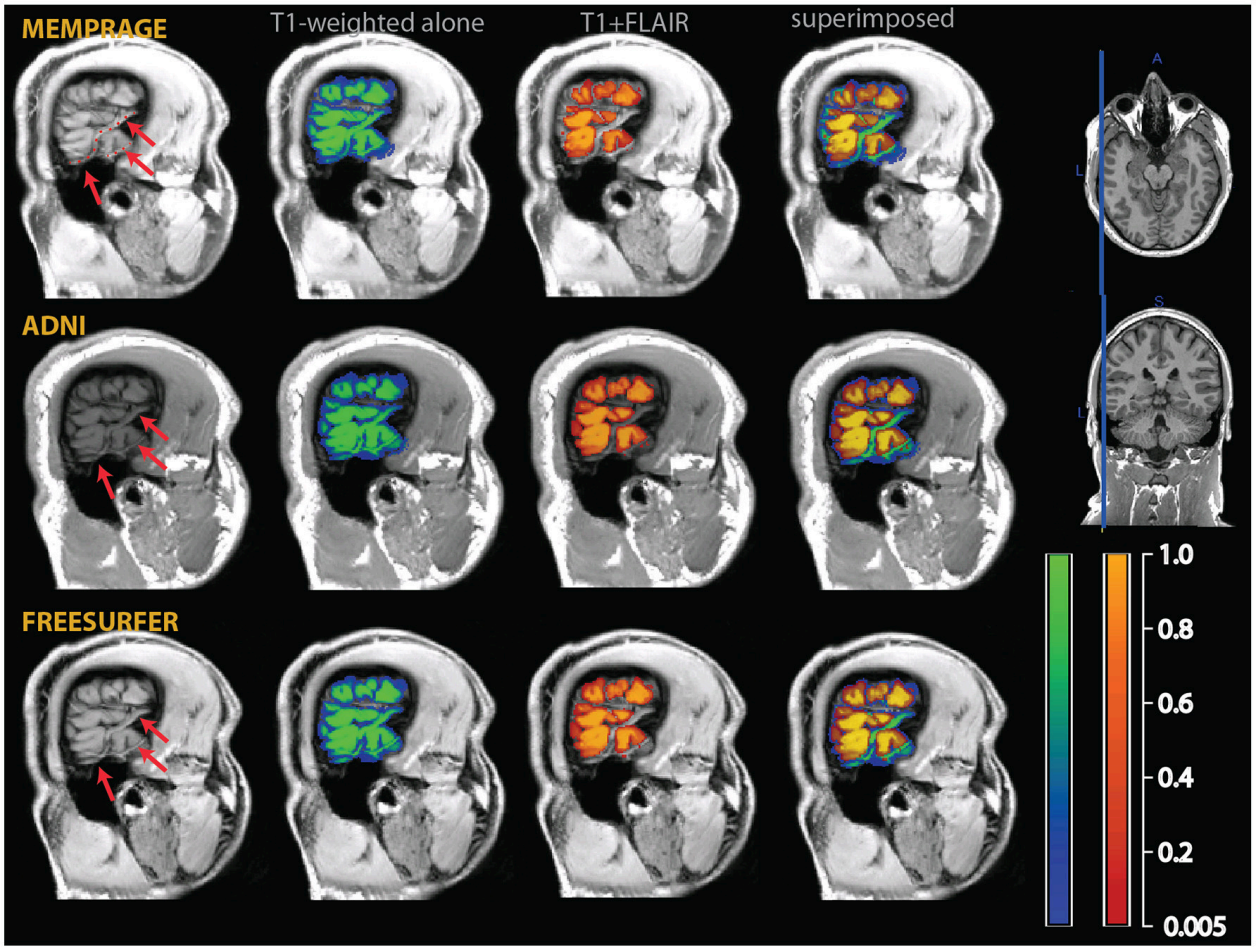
Example of a misclassification of sulcal vessels as gray matter (red arrows and dotted red line in the T1-weighted images on the left). As in the previous figures, the segmentation of the T1-weighted images alone is shown as a blue-green overlay, and the multimodal segmentation with the added FLAIR images in red-orange. Note the vessels misclassified as gray matter by the T1-weighted segmentation (in green in the rightmost column of images), but not in the multimodal procedure.
